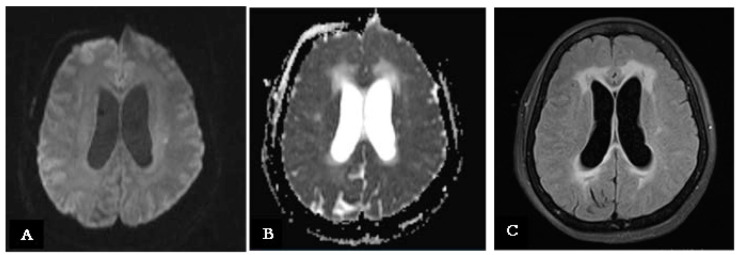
(A) A new MRI scan (performed during the stay in the infective disease ward) showing an hyperintensity signal on the left corona radiate on a DWI sequence, indicating a new ischemic lesion. (B) Same MRI brain scan on an ADC sequence showing a hypointense signal on the left corona radiate, indicating the same ischemic lesion on left corona radiate. (C) Same MRI brain scan on a FLAIR sequence showing a hyperintense signal on the left corona radiate, indicating the subacute evolution of the ischemic lesion.
